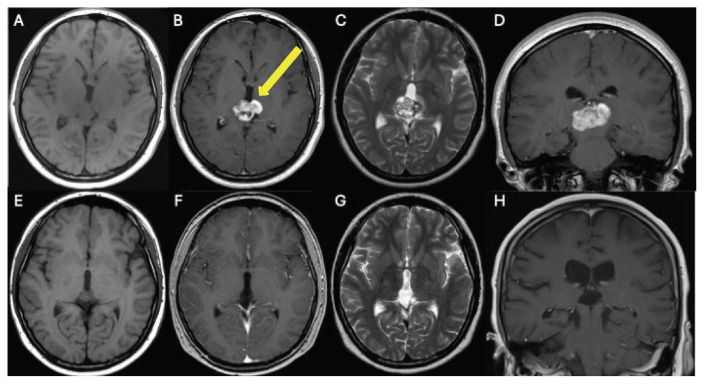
MRI of one patient with a pineal gland tumor. (A) Axial T1 sequence without contrast medium before treatment. An exophytic growth pattern of the tumor can be seen, there is no hydrocephalus recognizable. (B) Axial T1 sequence with contrast medium before radiation. (C) Axial T2 sequence with contrast medium before radiation. (D) Coronal T1 sequence with contrast medium before radiation. (E) Axial T1 sequence without contrast medium after radiation. (F) Axial T1 sequence with contrast medium after resection. (G) Axial T2 sequence with contrast medium after resection. (H) Coronal T1 sequence with contrast medium after resection.
